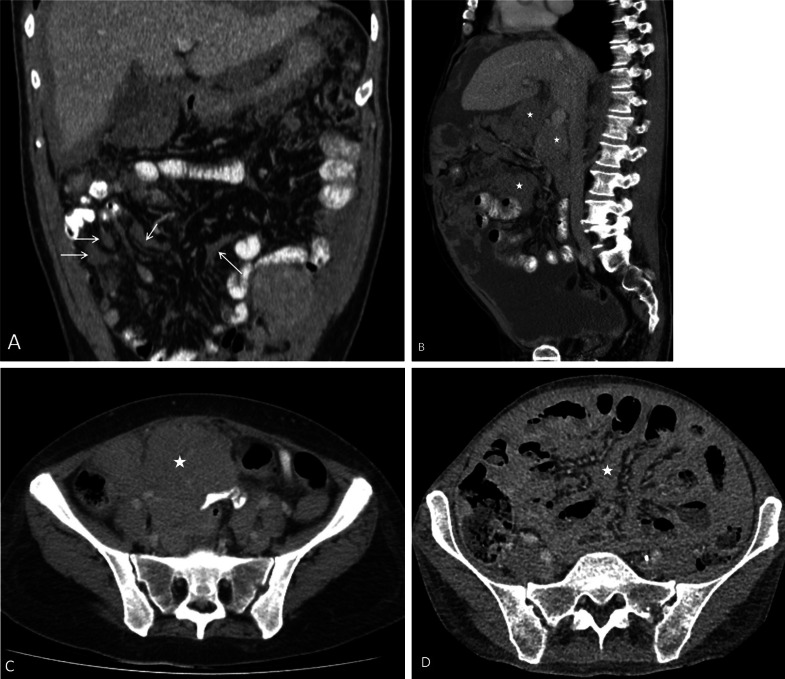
a–d CT images of different patients with malignant peritoneal mesothelioma showing findings of advanced mesenteric disease. a Nodular mesenteric fold thickening (arrows). b Sagittal CT showing soft tissue masses in the root of mesentery (*). c Mesenteric soft tissue mass (*) encasing small bowel. d Stellate mesentery on CT, which represents gross nodular thickening and retraction of the mesentery (*)
